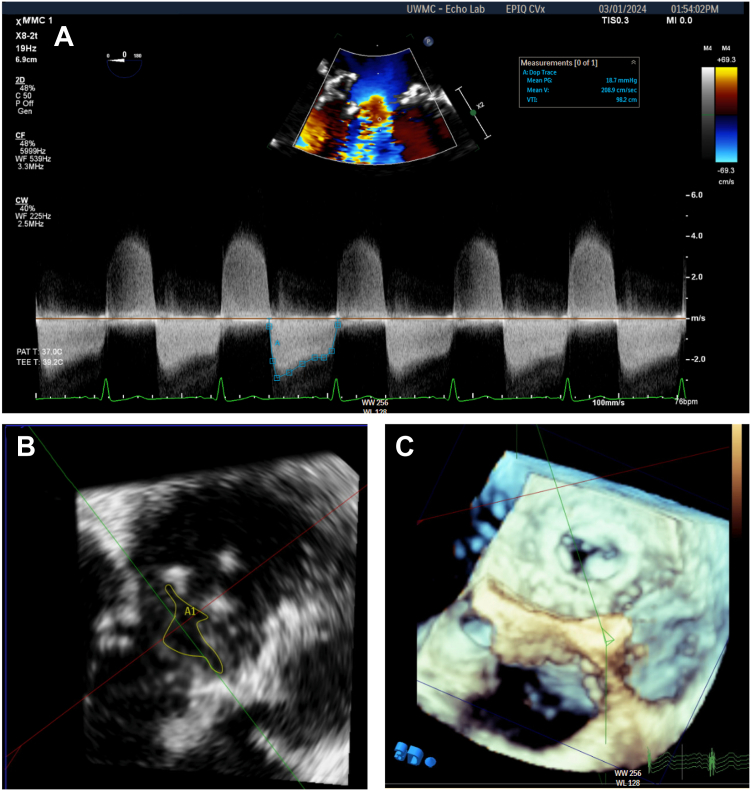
(A) Transesophageal echocardiogram before transcatheter mitral valve replacement demonstrating marked inflow acceleration across the bioprosthetic mitral valve (bioMVR). Mean gradient while under anesthesia of 18 mm Hg. (B) The 3 dimensional planimetry of the bioMVR. Valve area, 1.2 cm^2^. (C) The 3 dimensional image of severely stenotic bioMVR.
